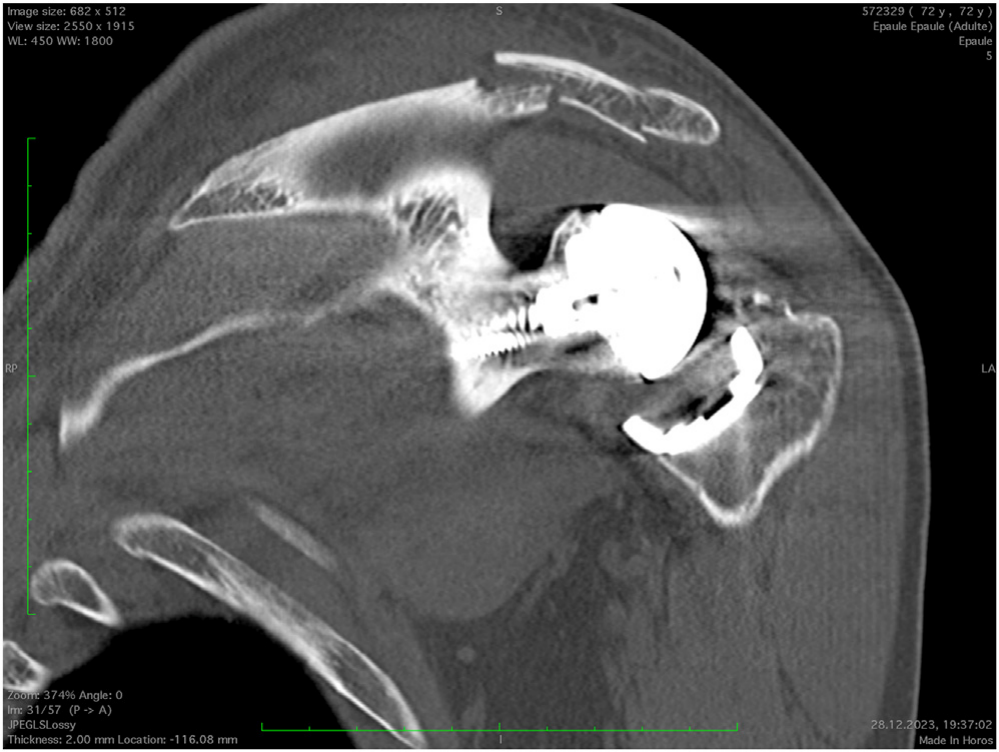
Coronal view of a left shoulder on computed tomography. Observe the displaced bifocal fracture of the scapular spine and the resulting inferior tilt of the acromion.
